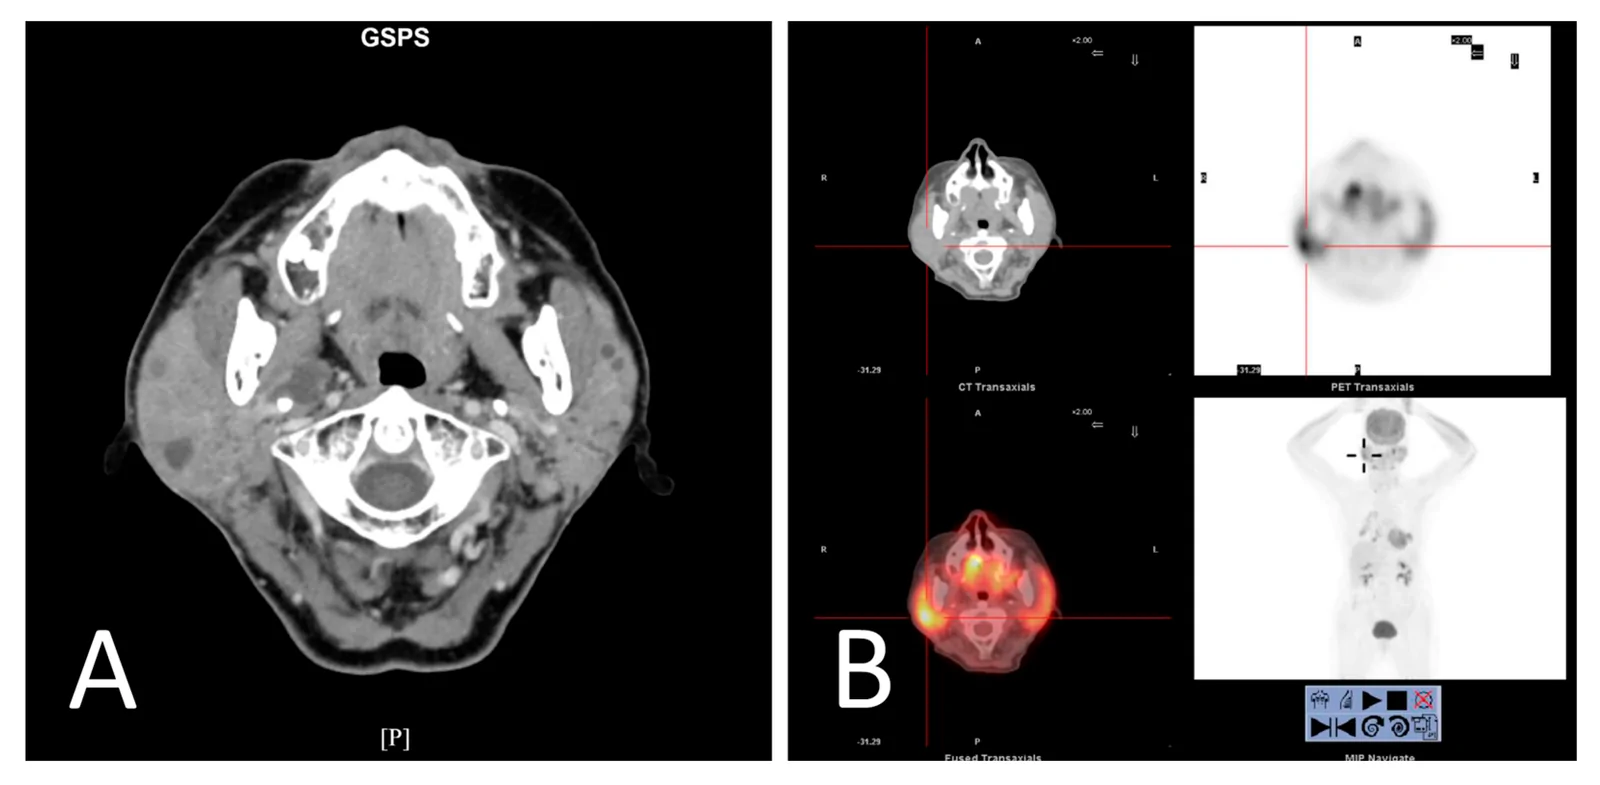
Subsequent neck computed tomography demonstrated bilateral parotid gland enlargement with multiple cystic changes and necrotic lymphadenopathy in the right parapharyngeal space (A), raising suspicion for lymphoma. The patient therefore underwent surgical excision of the right parotid lesion for definitive diagnosis. PET-CT demonstrated lymphoma involvement of bilateral parotid glands (B), multiple nodal regions above the diaphragm, and pulmonary lesions.
